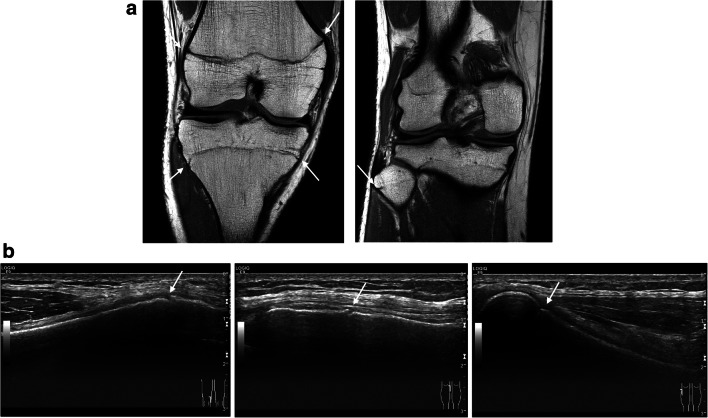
Right knee of a 17-year-old boy showing slightly open physeal plates (arrows) on a coronal T1-weighted MRI (a) and longitudinally oriented US images (b) on the medial distal femur (left image), the medial proximal tibia (middle image), and the proximal fibula (right image). On the US, note only the small gap between the metaphyseal and epiphyseal cortex, the physeal plate is not visible (US-S2)
